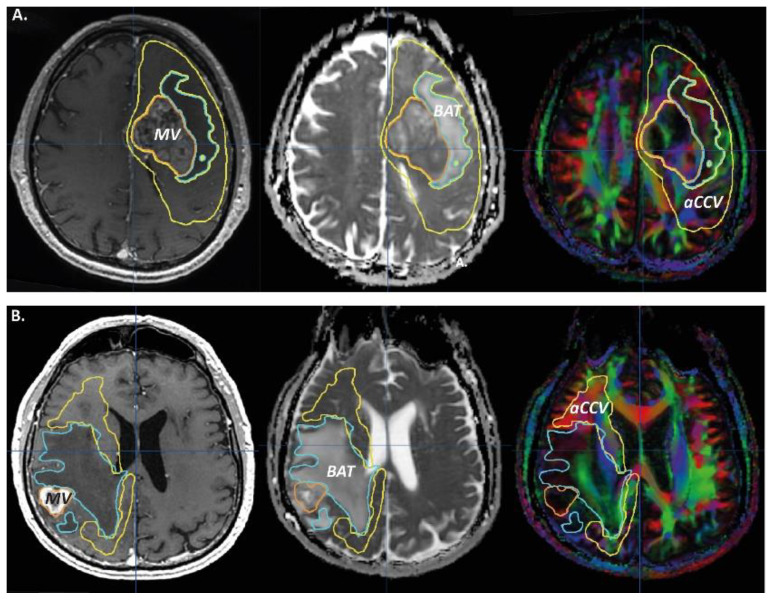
Manual contouring of the macroscopic volume (MV, orange), the BAT volume (blue) and the abnormal color-coded volume (aCCV, yellow) on axial MRI of GBM ((A); patient 11; left GBM) and metastasis ((B); patient 3; right metastasis) cases; left column, 3D T1-enhanced sequence; intermediate column, B0 diffusion weighted sequence; right column, color-coded DTI map.
